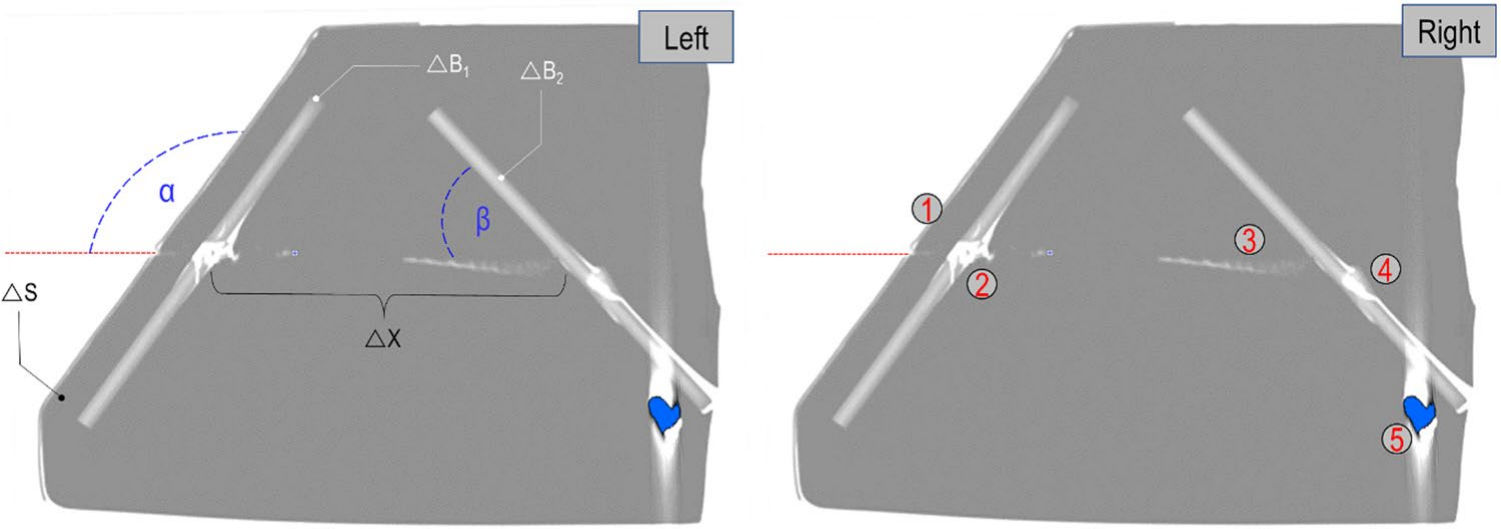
Axial view of the CT-scan images of the Model A.2. Left: representation of the shooting trajectory (red dotted line) as well the shooting parameters α (125°), △S (0.6 cm), △B_1_ (0.5 cm), △X (~ 11 cm), △B_2_ (0.5 cm), and β (43°) (for more details see Figs. 1 and 3). Right: the same CT-image as in A) with additional information: (1) entry hole, (2) hole in the first polyurethane wall with funnel form, (3) track in the gelatine attesting the side position of the projectile, (4) fracture in the second polyurethane wall, (5) position of the projectile after ricochet (in blue)
